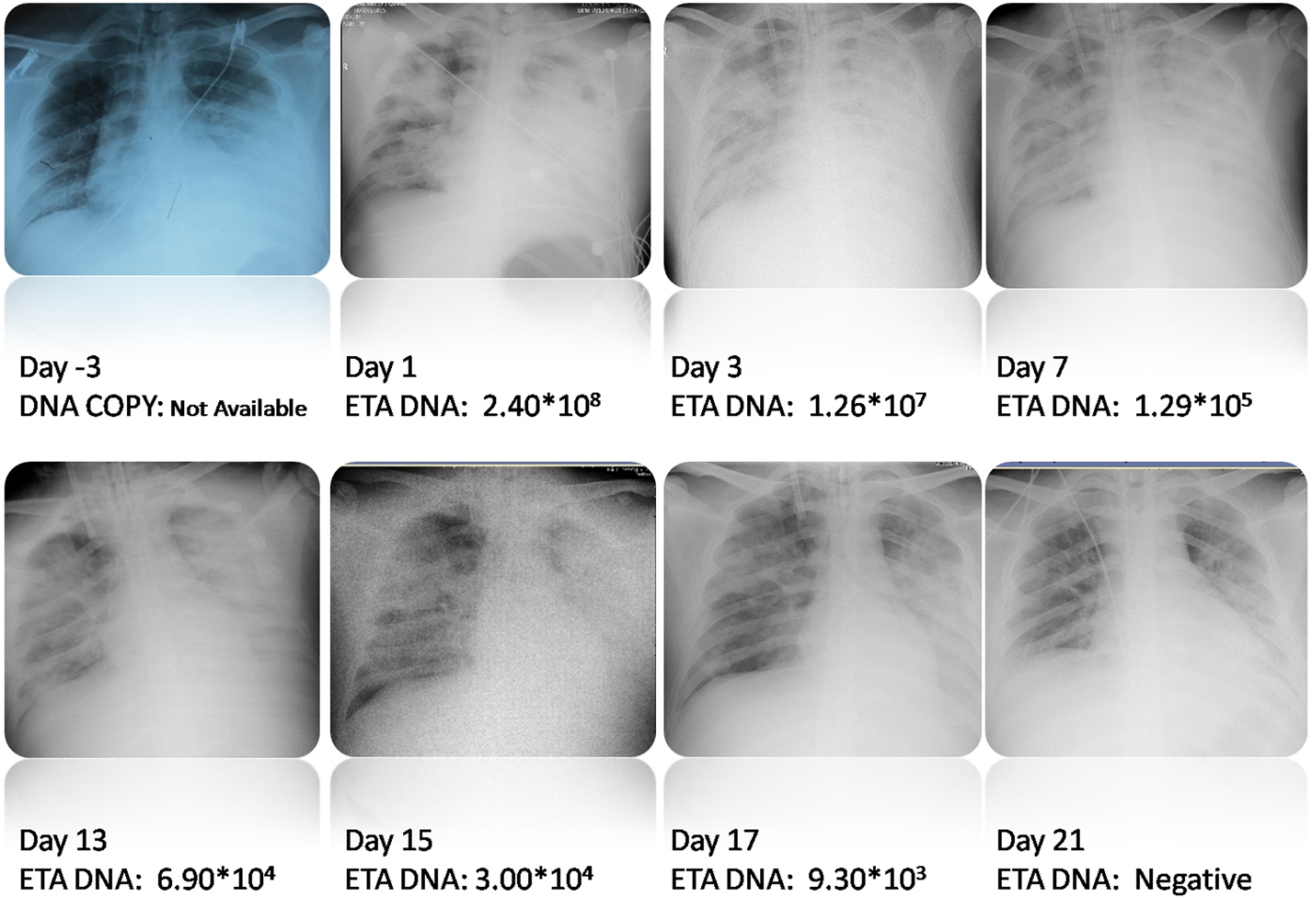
Radiographic findings and their relationship to viral load monitoring in patient 4. (Picture 1) Radiographs show widespread bilateral interstitial infiltrates at the onset of the disease. (Picture 2) The patient’s condition rapidly deteriorated within 3 days, and mechanical ventilation was required. (Picture 3) The patient’s adenoviral infection progressed, so supplementary oxygen was given on day 3 of admission. (Picture 4–8) Radiographs show gradual recovery with supportive therapy. Also shown are the trends of viral load detected with endotracheal aspirates (ETAs) and their relationship to radiological changes.
